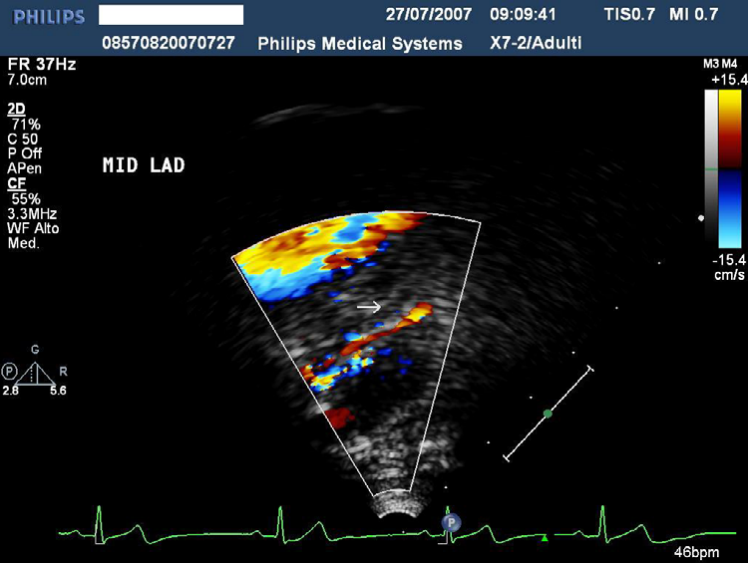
shows the ultrasound findings of proximal left anterior highlighted with color-Doppler.
